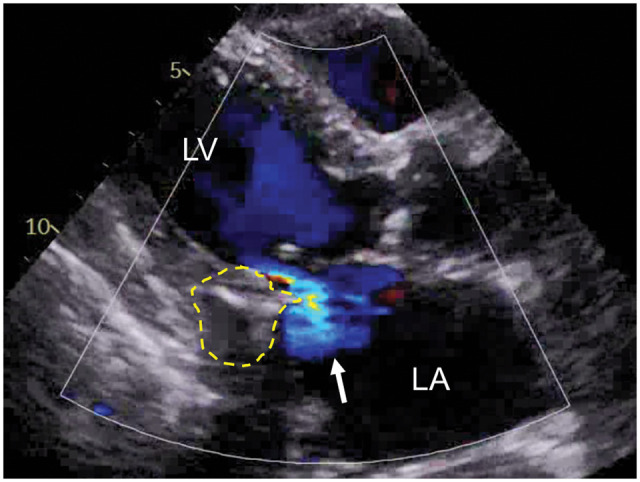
Transthoracic echocardiogram (parasternal long axis view). Preoperative echocardiogram demonstrating an internal echolucent mass with acoustic shadow (dashed line) and mitral valve insufficiency (arrow). LA: left atrium; LV: left ventricle
